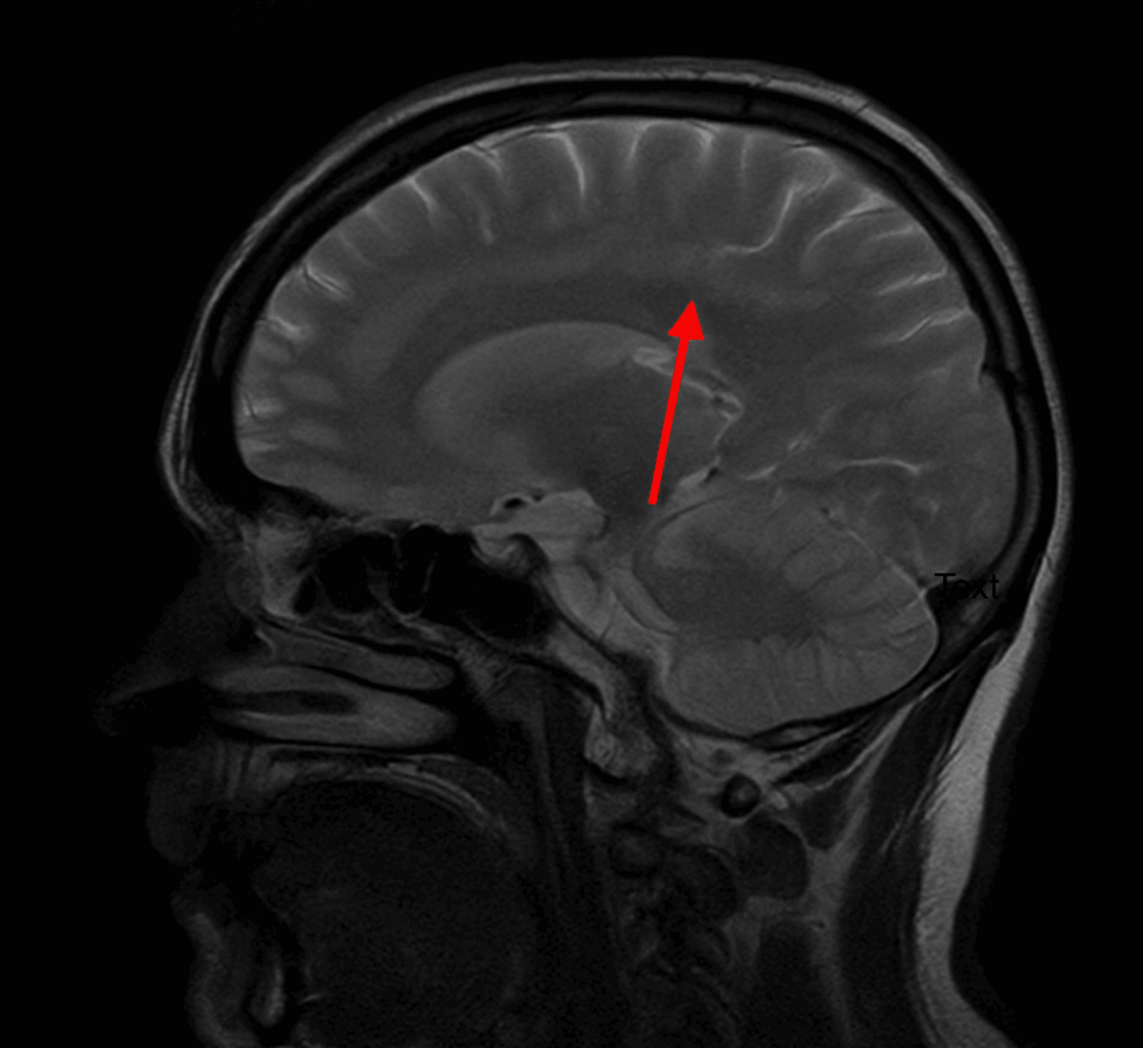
Sagittal T1-weighted MRI brain demonstrating complete agenesis of the corpus callosum with absence of callosal fibers and a high-riding third ventricle.
